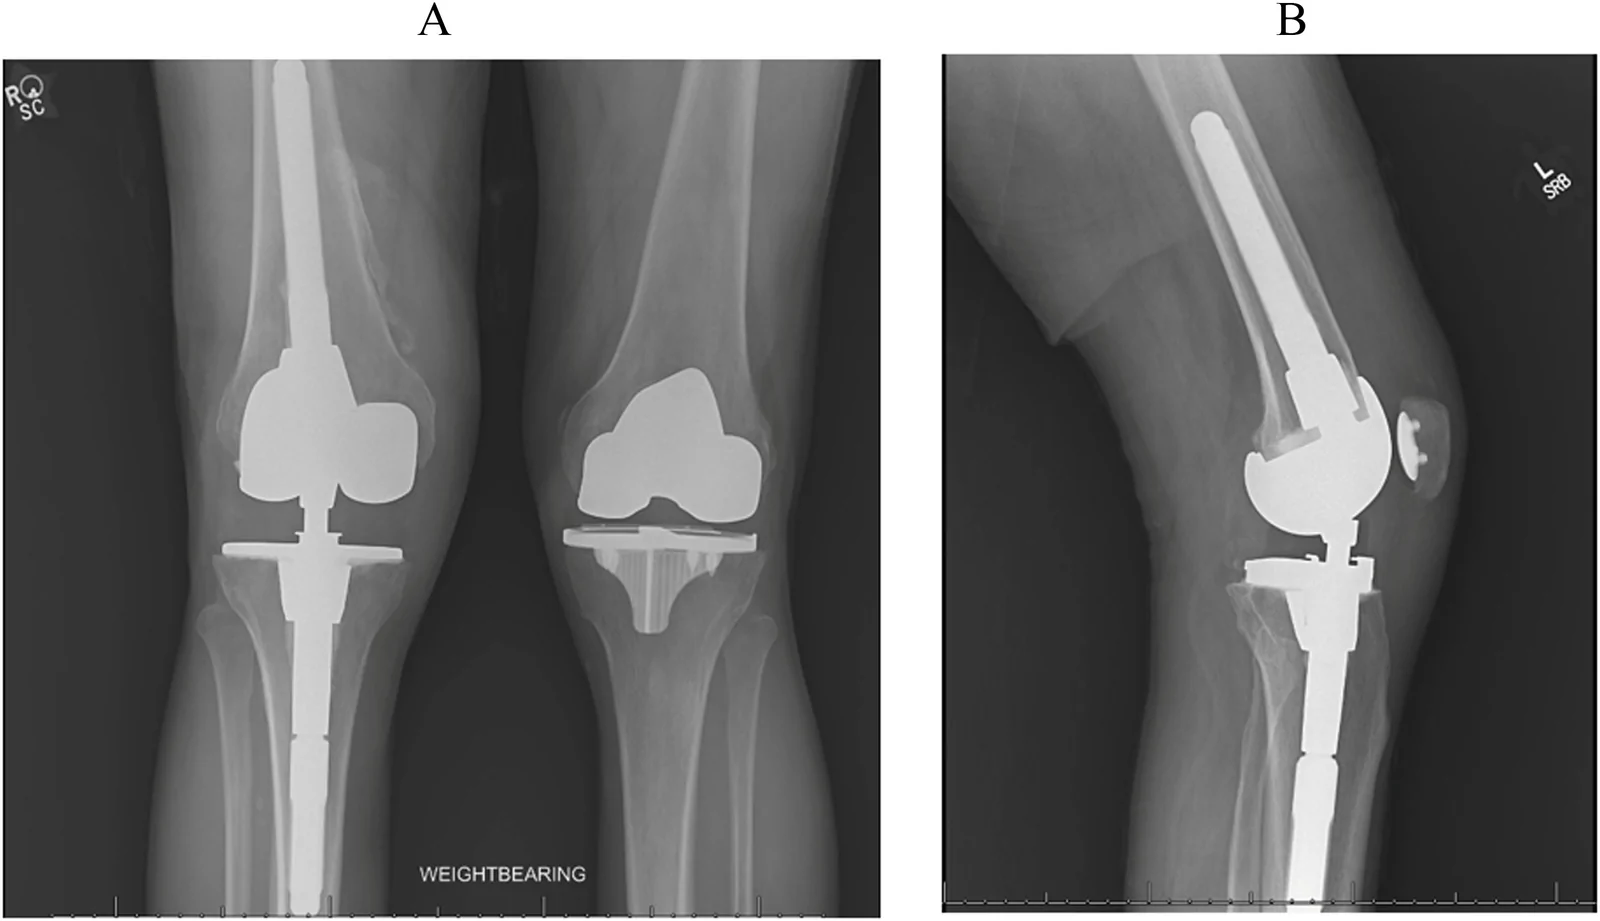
Postoperative radiographic evaluation of rotating-hinge knee arthroplasty. (A) Anteroposterior (B) Lateral weightbearing radiographs demonstrate a well-aligned Zimmer Rotating Hinge Knee prosthesis. Long press-fit stems provide diaphyseal stability, augmented by metaphyseal cone fixation to manage bone loss and guarantee rigid construct structural integrity following extensive soft tissue debridement.
